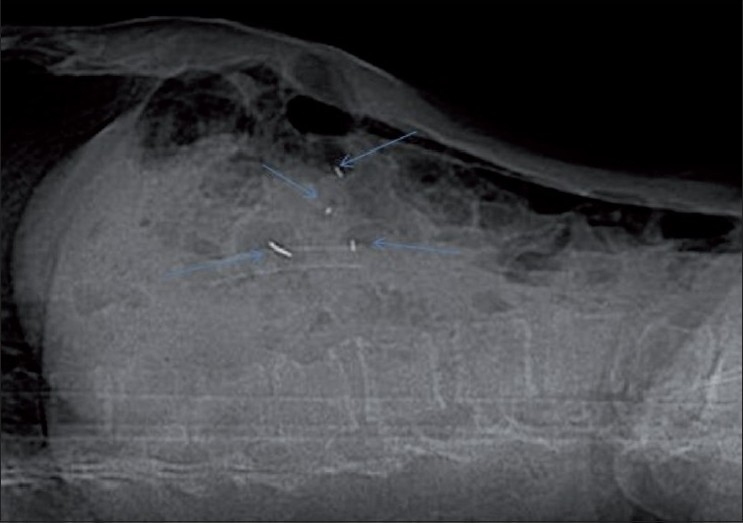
Lateral scanogram after placement of the fiducials under CT scan guidance, shows all the fiducials as well defined radiodense foci (arrows)
